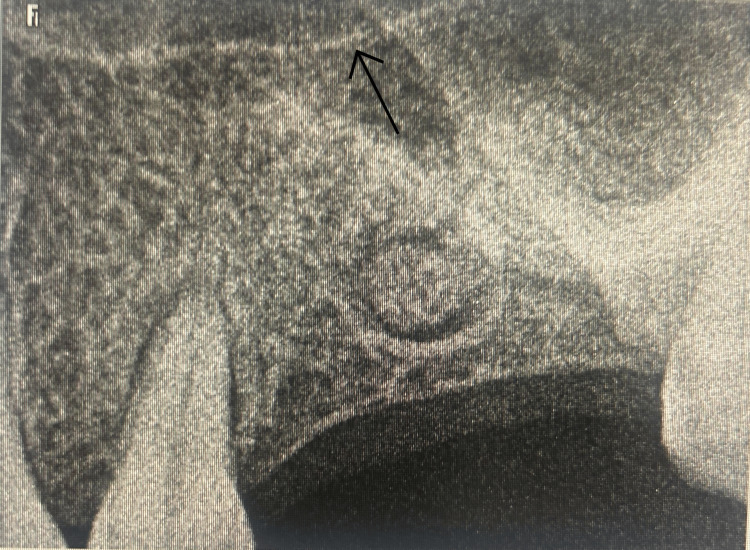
Post-healing IOPA demonstrating augmented bone height. The arrow indicates the lifted sinus membrane. IOPA: intraoral periapical radiograph
